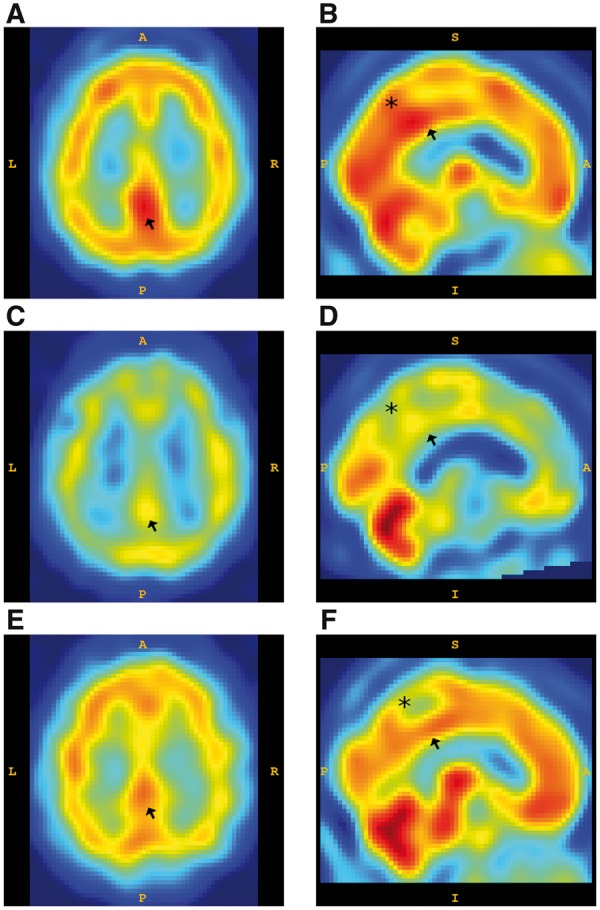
^99m^Tc-HMPAO SPECT perfusion images from control, Alzheimer disease, and dementia with Lewy bodies donors. Representative axial slice images (A, C, E) through the posterior cingulate and (B, D, F) midline sagittal slice images of perfusion determined using ^99m^Tc-HMPAO SPECT. In older normal patients (A, B) without dementia, the posterior cingulate cortex (arrow) and precuneus (asterisk) appear relatively bright. In AD patients (C, D), perfusion intensity is reduced in both these structures. In DLB patients (E, F), the precuneus shows a greater reduction compared with the posterior cingulate cortex, which shows relatively preserved perfusion. (L, left; R, right; A, anterior; P, posterior; S, superior; I, inferior.)
